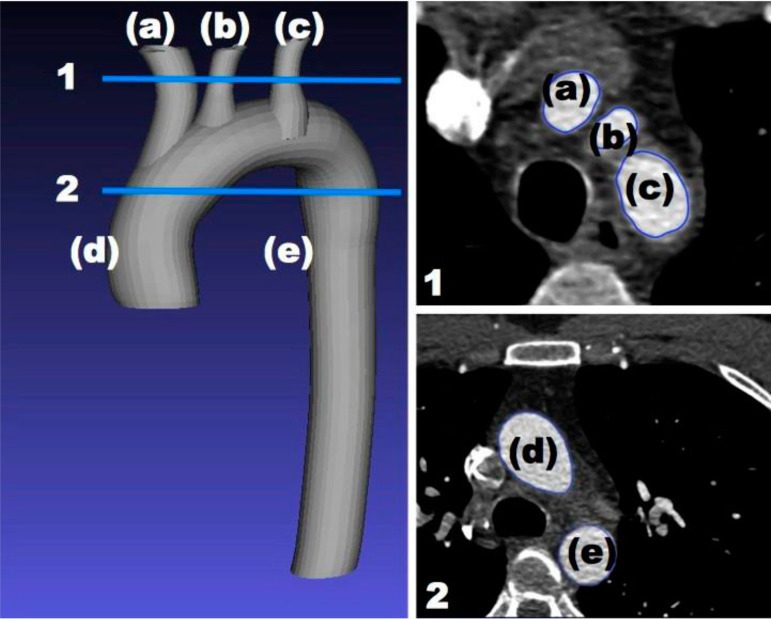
Substructures of the heart model in this study shown in relation to two slices out of the 400 slices of computed tomography scans. The structures are the ascending aorta (d), descending aorta (e), subclavian artery (a), left common carotid artery (b), and brachiocephalic artery (c). The two slices were captured on a two-dimensional plane marked with horizontal lines (1&2).
